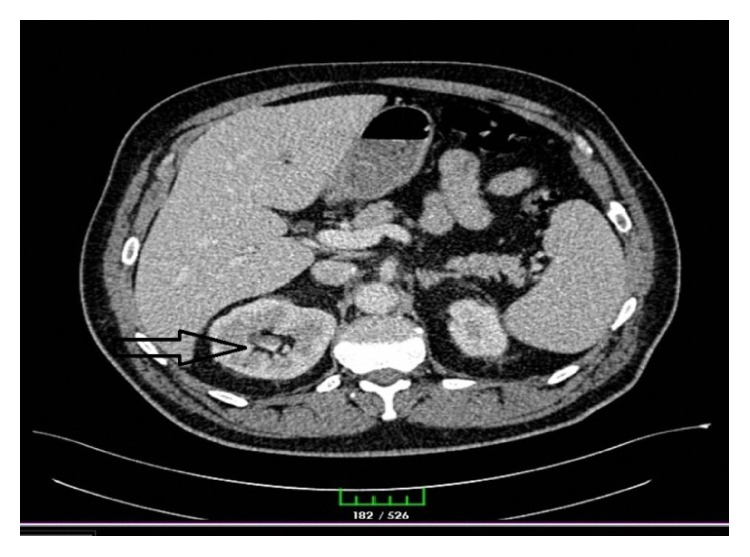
CT image showing hydronephrosis in the right kidney.
